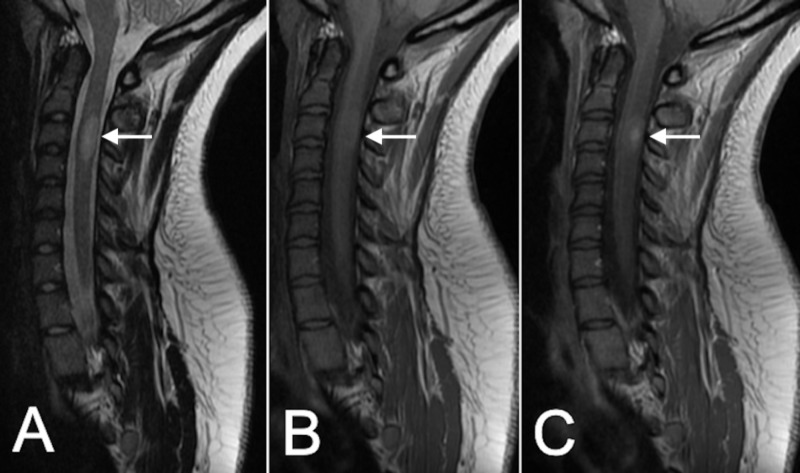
Initial MRI Presentation MRI shows a C3 cervical intramedullary lesion (arrow) that is hyperintense on T2-weighted images (A), isointense on T1-weighted images (B), with areas of contrast enhancement (C).
